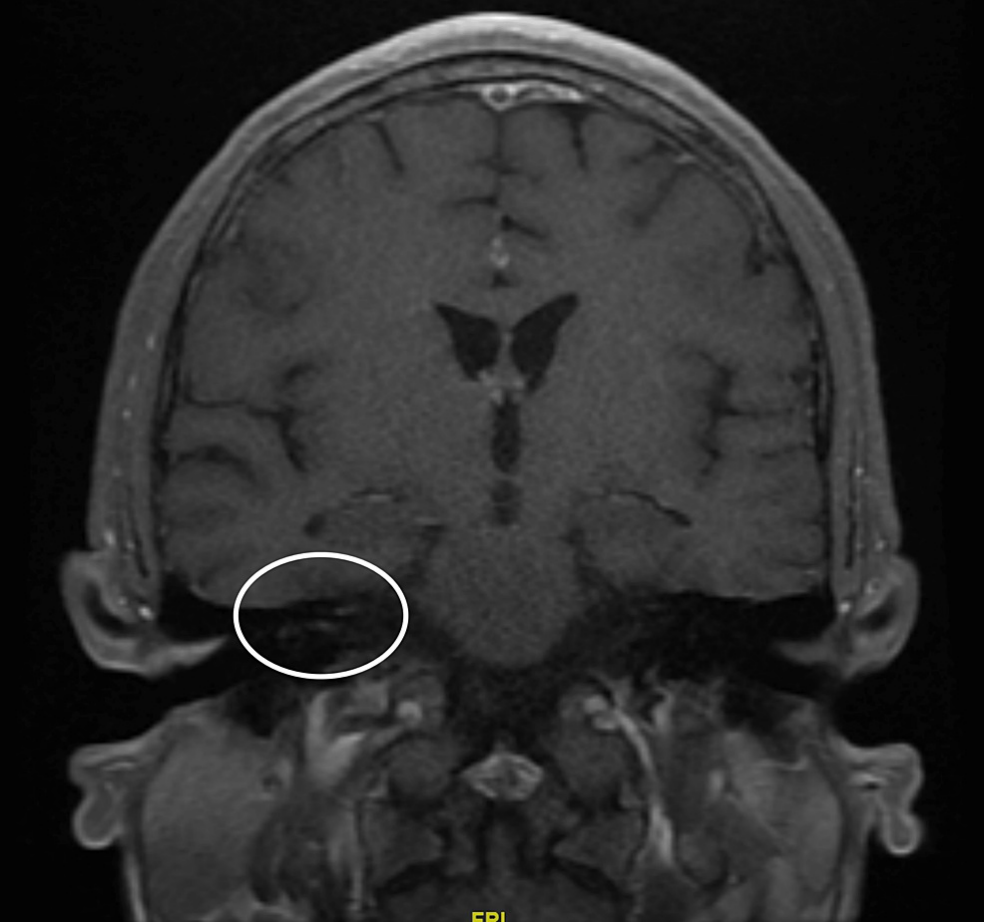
Brain MRI with and without contrast showing a punctate focus of enhancement in the distal right internal auditory canal
